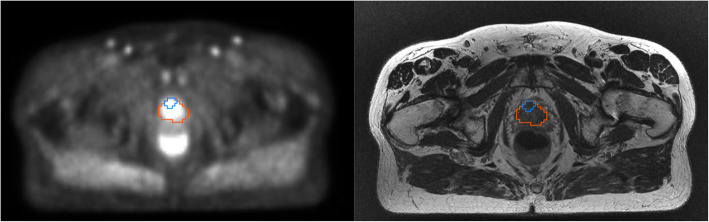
Lesions for one patient on PET and T2-weighted MRI. The prostate (red) and hotspot (blue) were drawn by specialists in oncology as a part of radiotherapy treatment planning, based on multiple MR sequences and PET images
